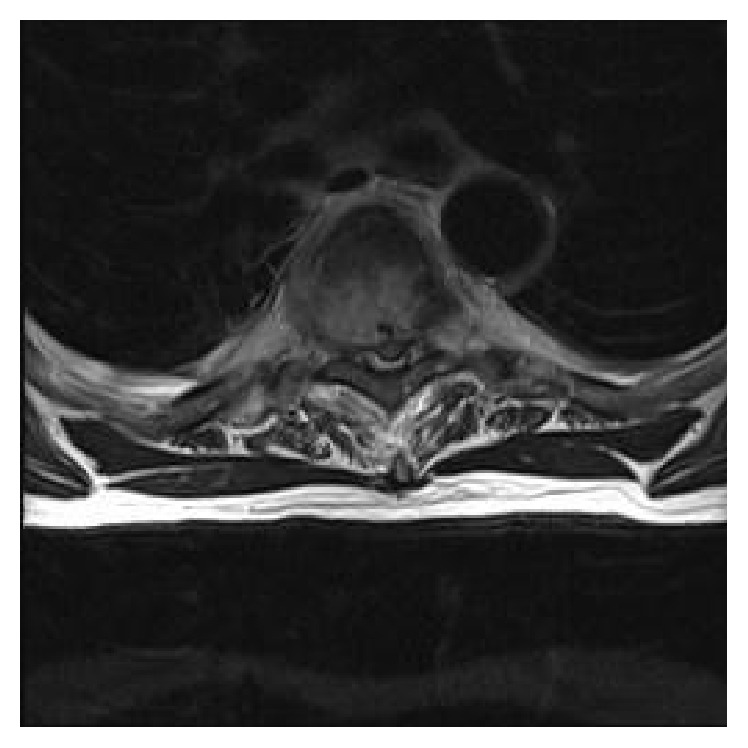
MRI scan, axial T1 weighted image, showing posterior soft tissue mass at the level of T5 with associated pathological fracture and diffuse paravertebral oedema. There is evidence of moderate compression of the spinal cord.
